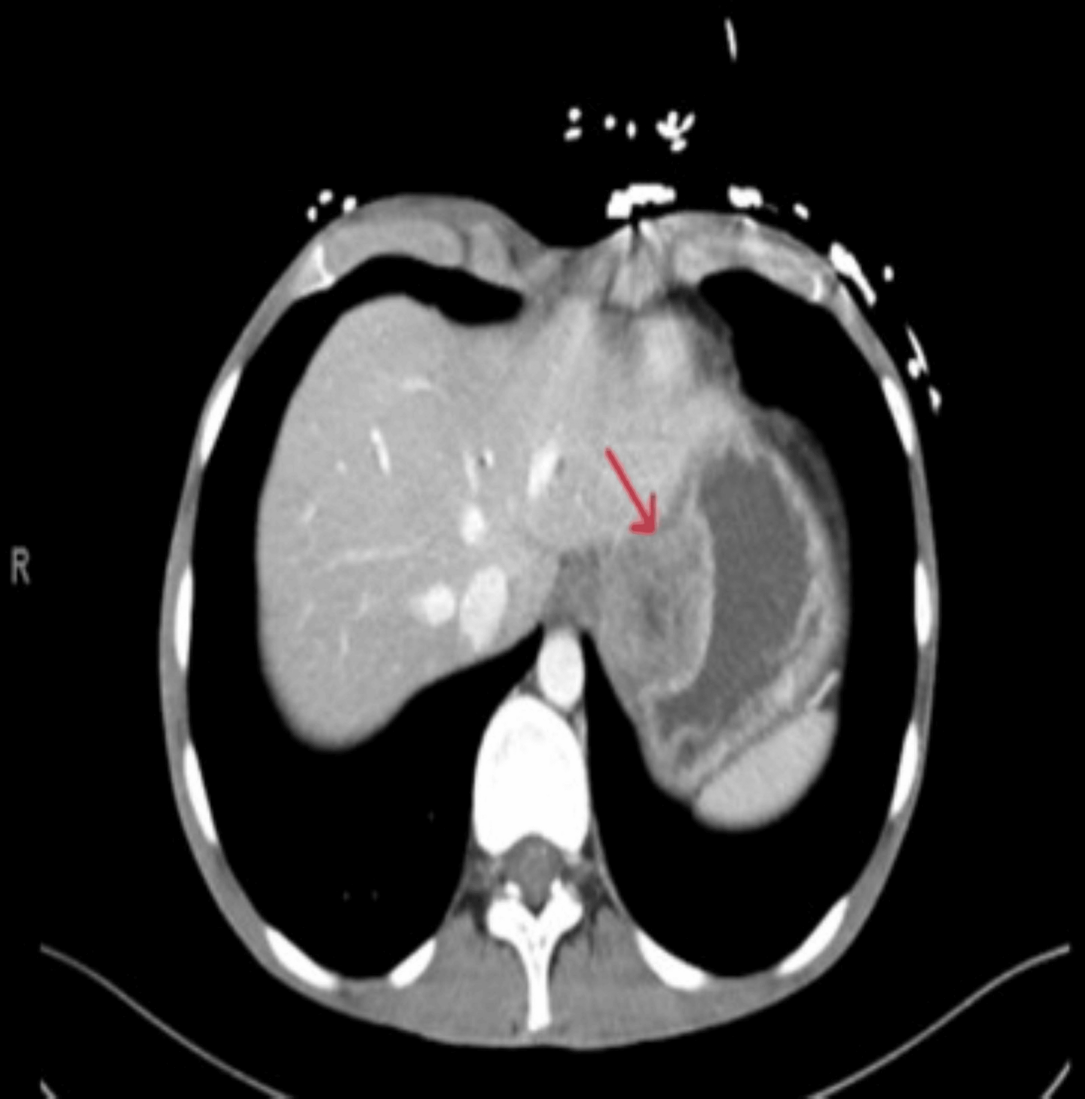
CT of the abdomen revealing a mass in gastric cardia (red arrow)
